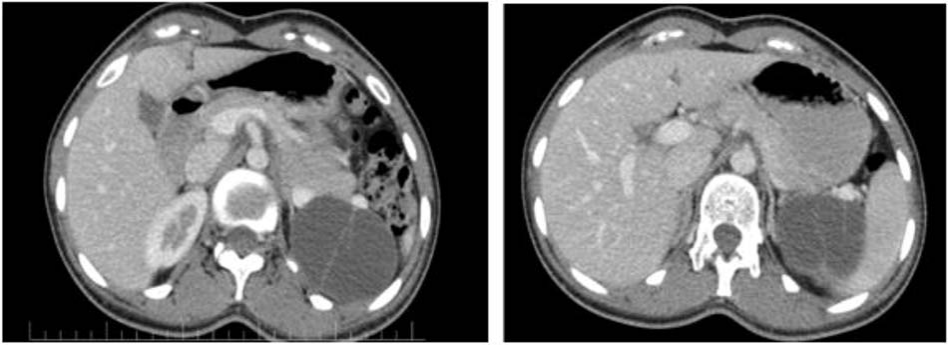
The pancreatic cystic mass appearance on the patient’s CT scan.
